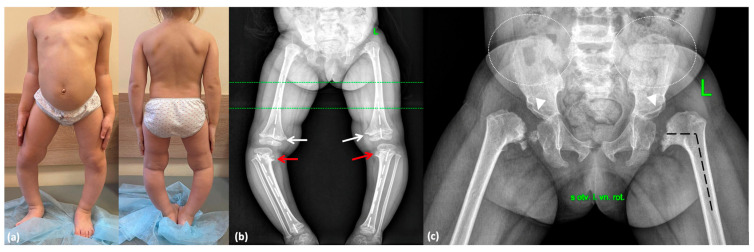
Phenotype and skeletal radiographies of P3 at various ages: (a) A photo taken at 3 years 11 months. (b) Severe varus deformity (white broken lines) secondary to the Blount-like tibia vara (red arrows) and metaphyseal irregularities predominantly in the medial parts of the femur (white arrows) at 3.5 years. (c) Coxa vara (black broken lines), abnormal ossification of the femoral neck, dysplastic acetabulae (white arrowheads), and lace-like appearance of the iliac crests (indicated by white dotted lines) at 3 years and 5 months.
